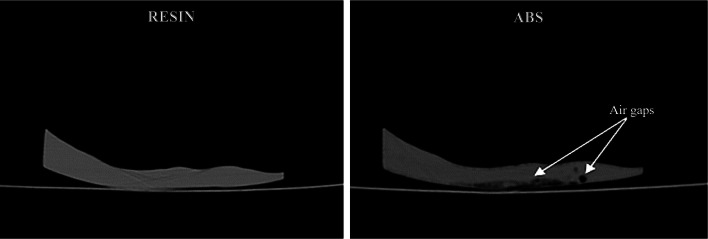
CT images of the Resin and ABS samples acquired with a tube voltage of 120 kV, current of 410 mA, and a slice thickness of 1.25 mm
